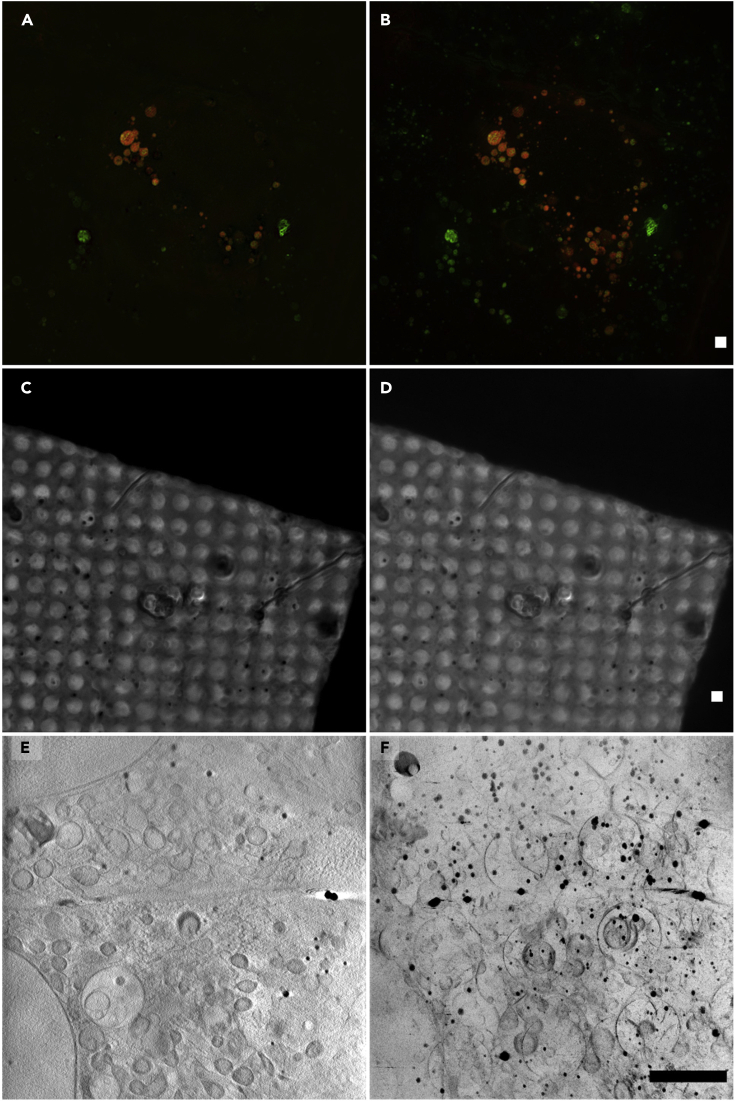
Slices from data z-stacks compared to their contrast-adjusted 2D maximum/minimum intensity projections The projection images demonstrate the highlighted information for registration. (A and B) (A) Slice from an SIR image stack and its total data maximum intensity projection (B). (C and D) (C) Slice from a bright-field z-stack taken on the cryoSIM and its total data minimum intensity projection (D). (E and F) (E) Slice from an X-ray tomogram and the corresponding minimum intensity projection of all data slices (F). The scale bars are 2 μm.c.Choose minimum as the projection type for the bright-field image stack and the X-ray tomogram stacks (Figures 3C–3F).d.Manually adjust the contrast using the minimum and maximum sliders on the histogram in the bar on the right in Icy to obtain the best contrast for viewing features in the image.e.Save these projection images to use in the next steps.
